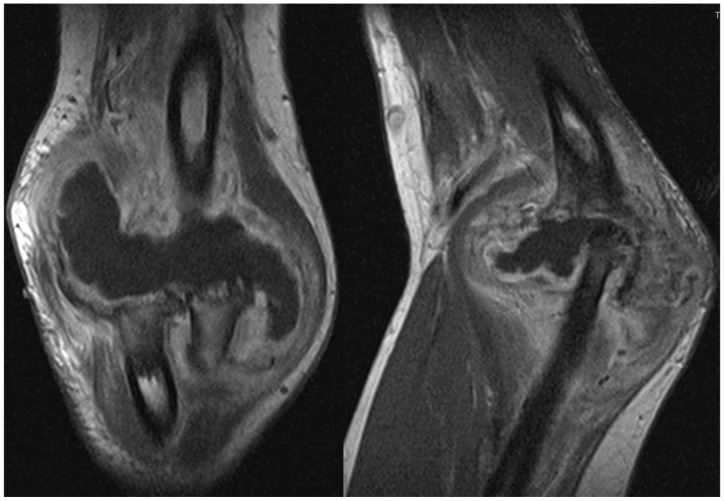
Magnetic resonance imaging (MRI) coronal and sagittal T1 weight views of the right elbow. Involvement of periarticular soft tissue with a large mass through the articulation.
